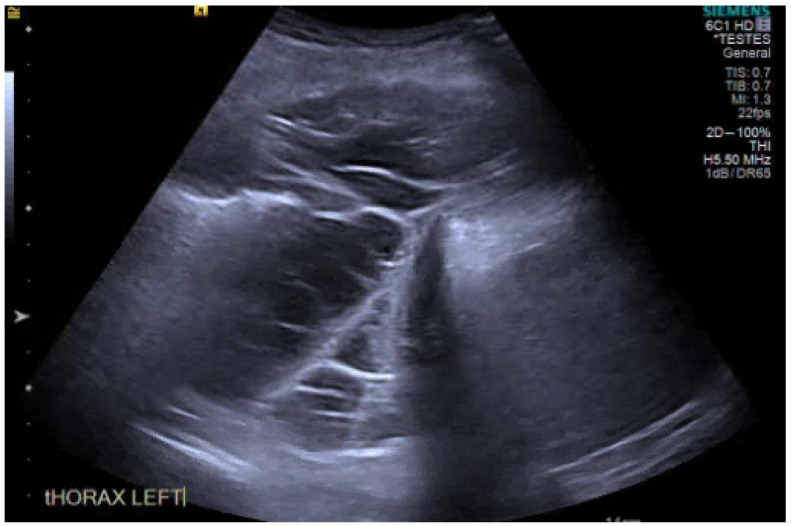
Thoracic ultrasound (TUS) image of a septated effusion.
